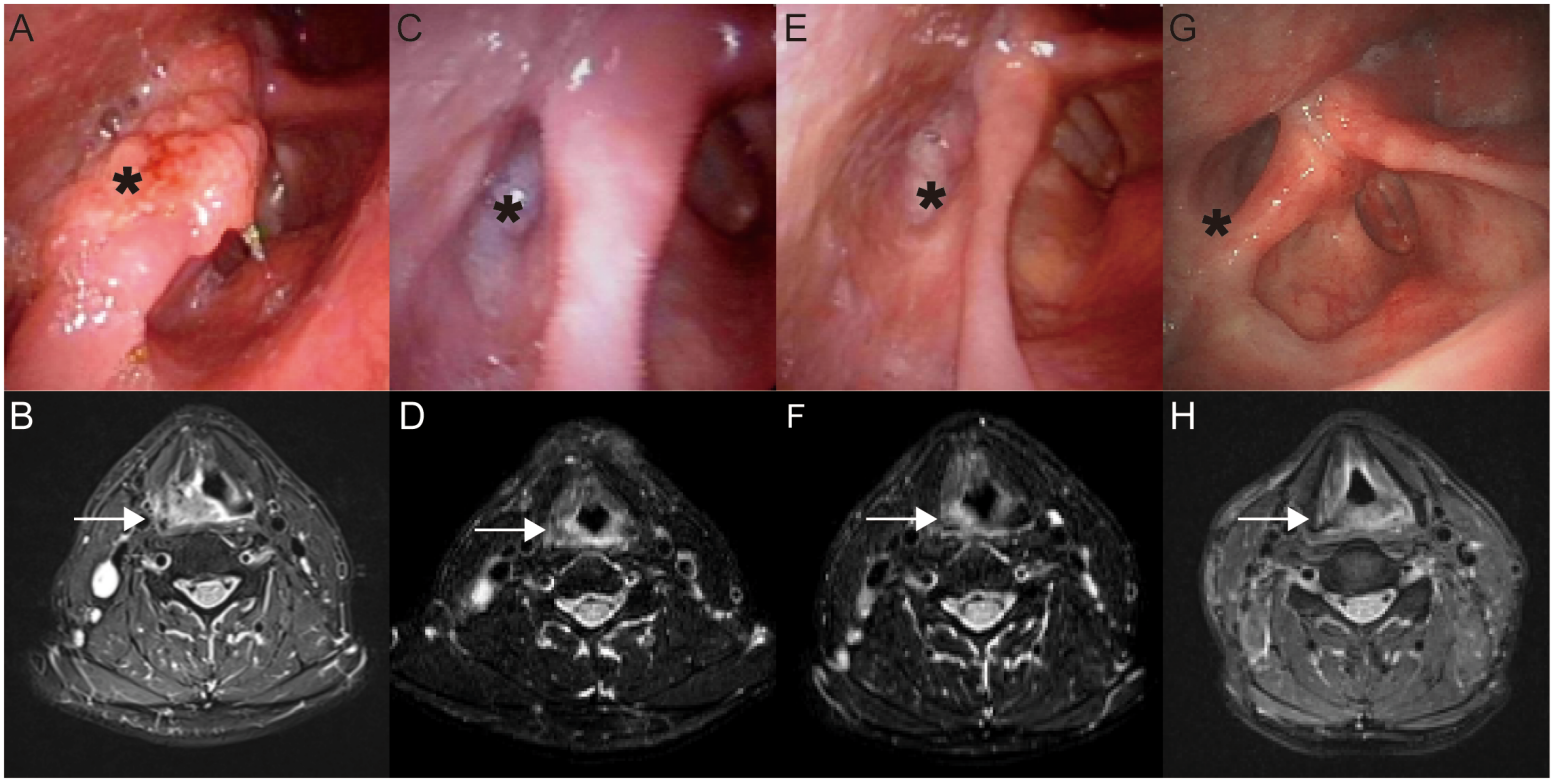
A representative case of neoadjuvant chemoimmunotherapy. A 56-year-old male patient was diagnosed with hypopharyngeal squamous cell carcinoma (T2N2bM0, stage IVA, AJCC staging). Pre-treatment laryngoscopy (A) revealed a cauliflower-like neoplasm at the lateral margin of the right arytenoid and right pyriform sinus (indicated by a black asterisk). MRI of the larynx (B) demonstrated a mass (22 mm × 16 mm) in the right pyriform sinus-supraglottic region (indicated by a white arrow), accompanied by multiple enlarged lymph nodes (42 mm × 16 mm) on the right side. The patient was subjected to neoadjuvant therapy with pembrolizumab (200 mg, day 1), paclitaxel (135 mg/m², day 2), and cisplatin (35 mg/m², days 2–4). Follow-up laryngoscopy (C) after the first cycle of neoadjuvant therapy showed mucosal hyperemia with minimal effusion in the right pyriform sinus (indicated by a black asterisk). MRI (D) revealed a reduction in the primary lesion to approximately 14 mm × 8 mm (indicated by a white arrow), with lymph node size decreasing to 14 mm × 11 mm. After the second cycle, laryngoscopy (E) demonstrated mucosal roughness in the right pyriform sinus (indicated by a black asterisk), while MRI (F) showed further regression of the primary lesion to 12 mm × 8 mm (indicated by a white arrow), with the largest lymph node diameter reduced to 7 mm. Imaging assessment indicated a PR. The patient subsequently underwent transoral resection of the right pyriform sinus lesion and right cervical lymph node dissection. Postoperative pathology revealed lymphocyte and plasma cell infiltration in the primary site with no residual tumor, while lymph nodes exhibited chemotherapy-induced changes without evidence of malignancy, achieving a pCR. Adjuvant radiotherapy was administered postoperatively. During regular outpatient follow-up, laryngoscopy (G) and MRI (H) at 19 months post-surgery showed no evidence of tumor recurrence or residual disease.
